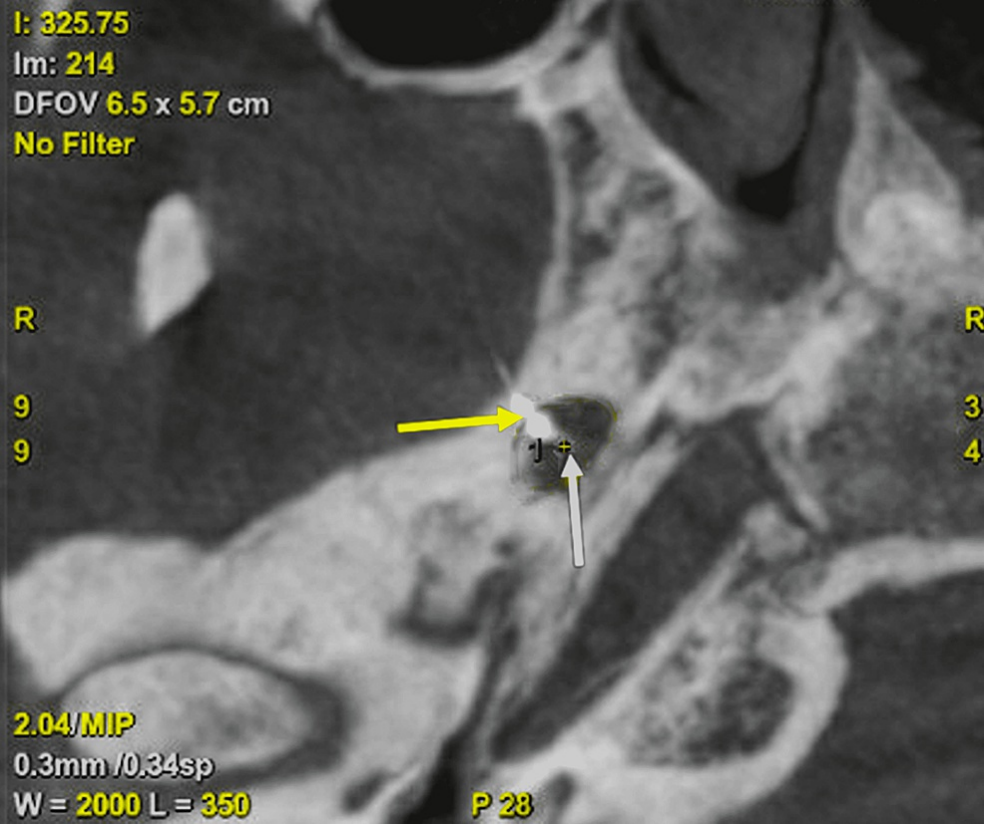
Cone beam CT skull for needle confirmation Insertion of needle tip identified (yellow arrow) through foramen ovale into Meckel’s cave (white arrow), confirming proper placement.
